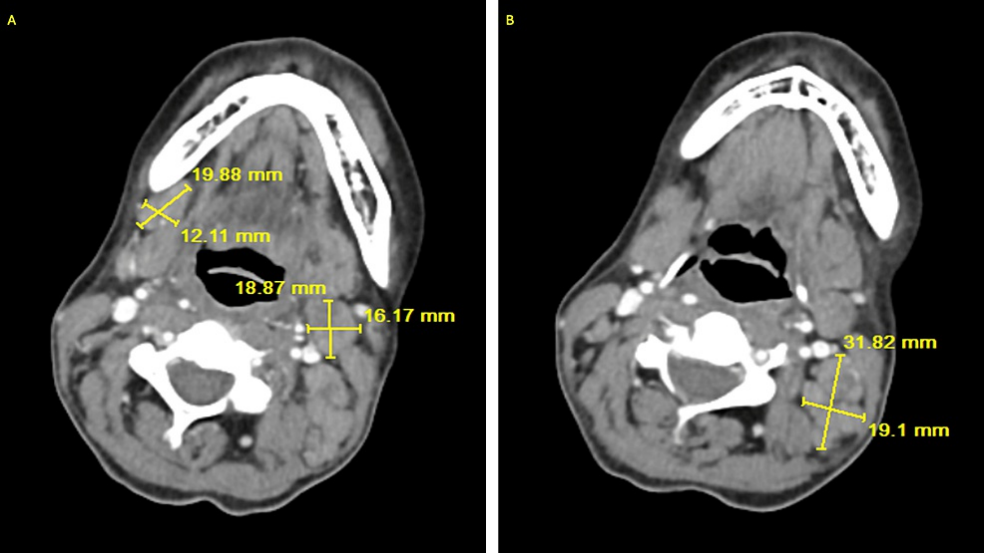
Computed tomography imaging of the soft tissue of the neck with marked cervical lymphadenopathy
